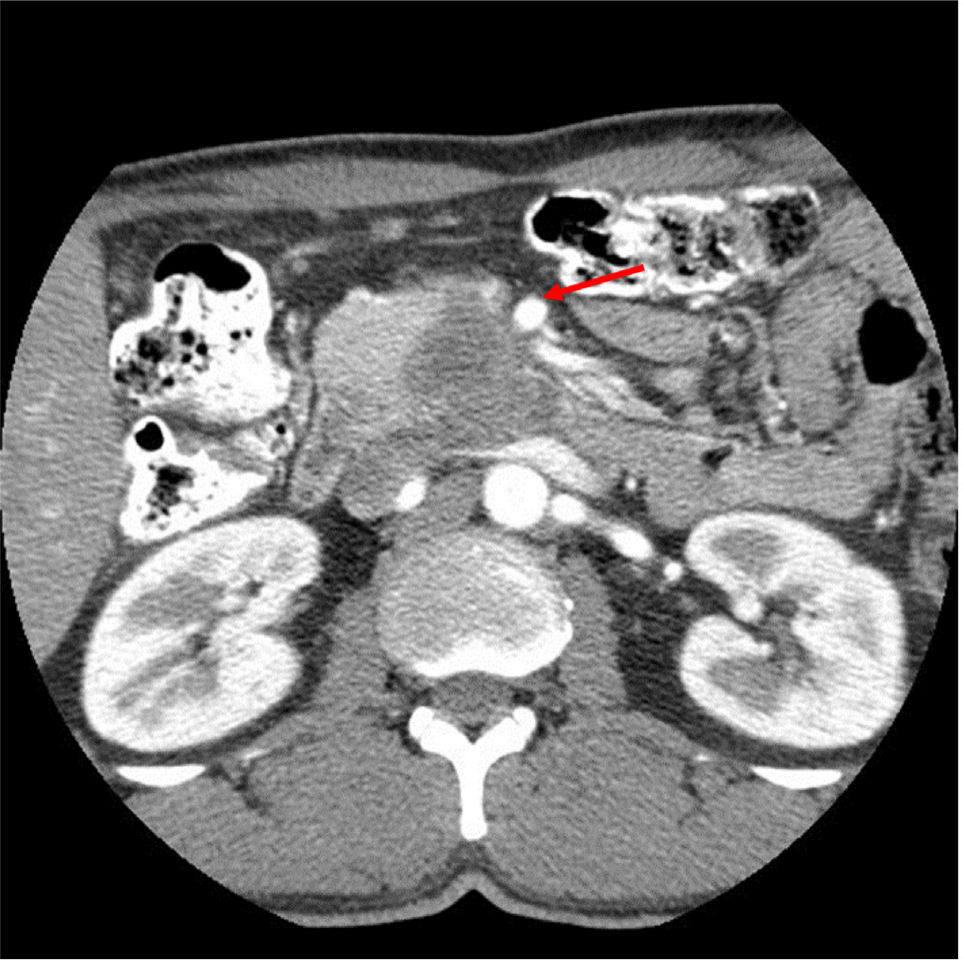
CT abdomen at initial work-up that shows a hypo-attenuated mass in the region of the uncinate process contacting 50% of the superior mesenteric vein, and approximately 25% of the superior mesenteric artery (see arrow) as well as contacting the left renal vein.
